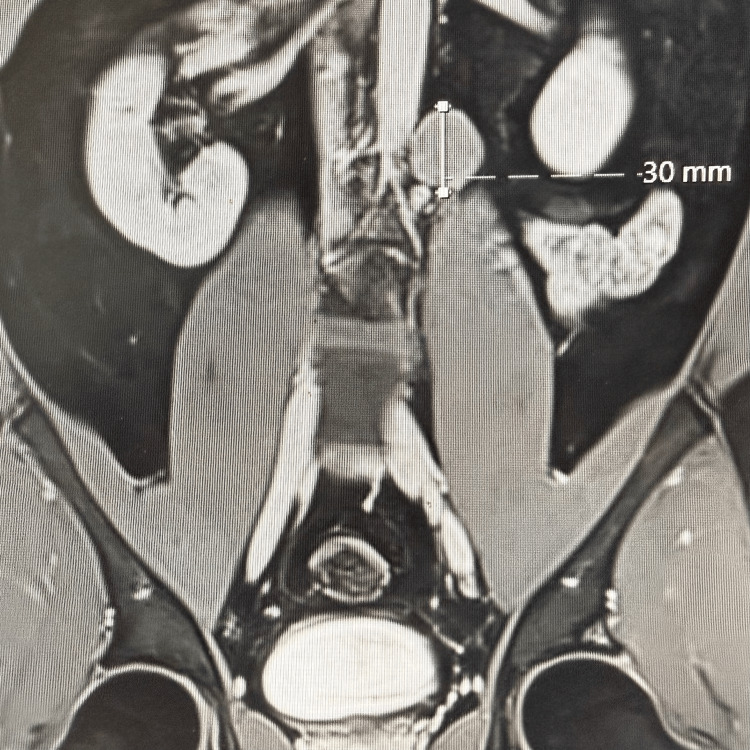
Preoperative MRI showing the location of the 3 cm para-aortic lymphadenopathy.
